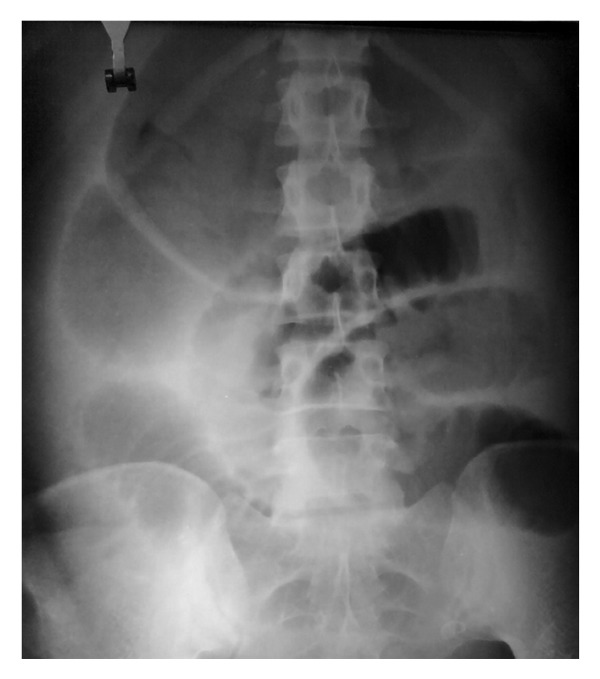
Plain abdominal radiograph with dilated colon.
